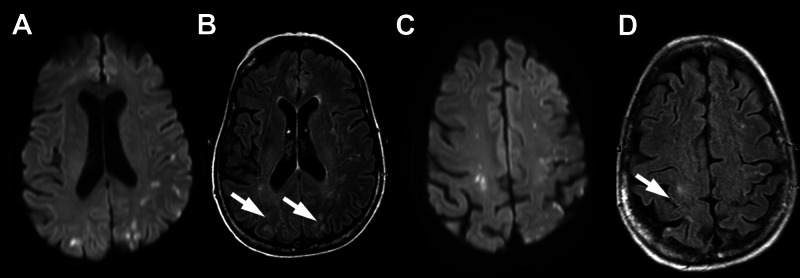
Brain MRI on the day of admission Diffusion-weighted sequences (A and C) demonstrate numerous cortical and subcortical foci of restricted diffusion in frontal and parietal lobes. On the FLAIR sequences (arrows B and D), these foci are only mildly hyperintense, consistent with acute embolic infarcts involving the anterior circulation. MRI: magnetic resonance imaging; FLAIR: fluid-attenuated inversion recovery
